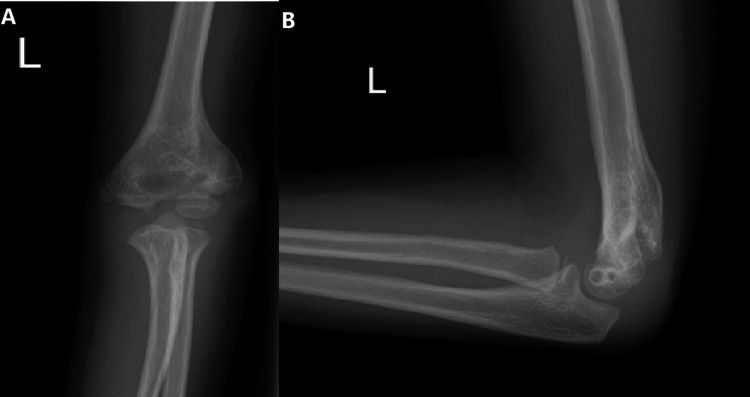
(A) Anteroposterior and (B) lateral radiographs demonstrating a union at 16 months post-injury (Case 1)
